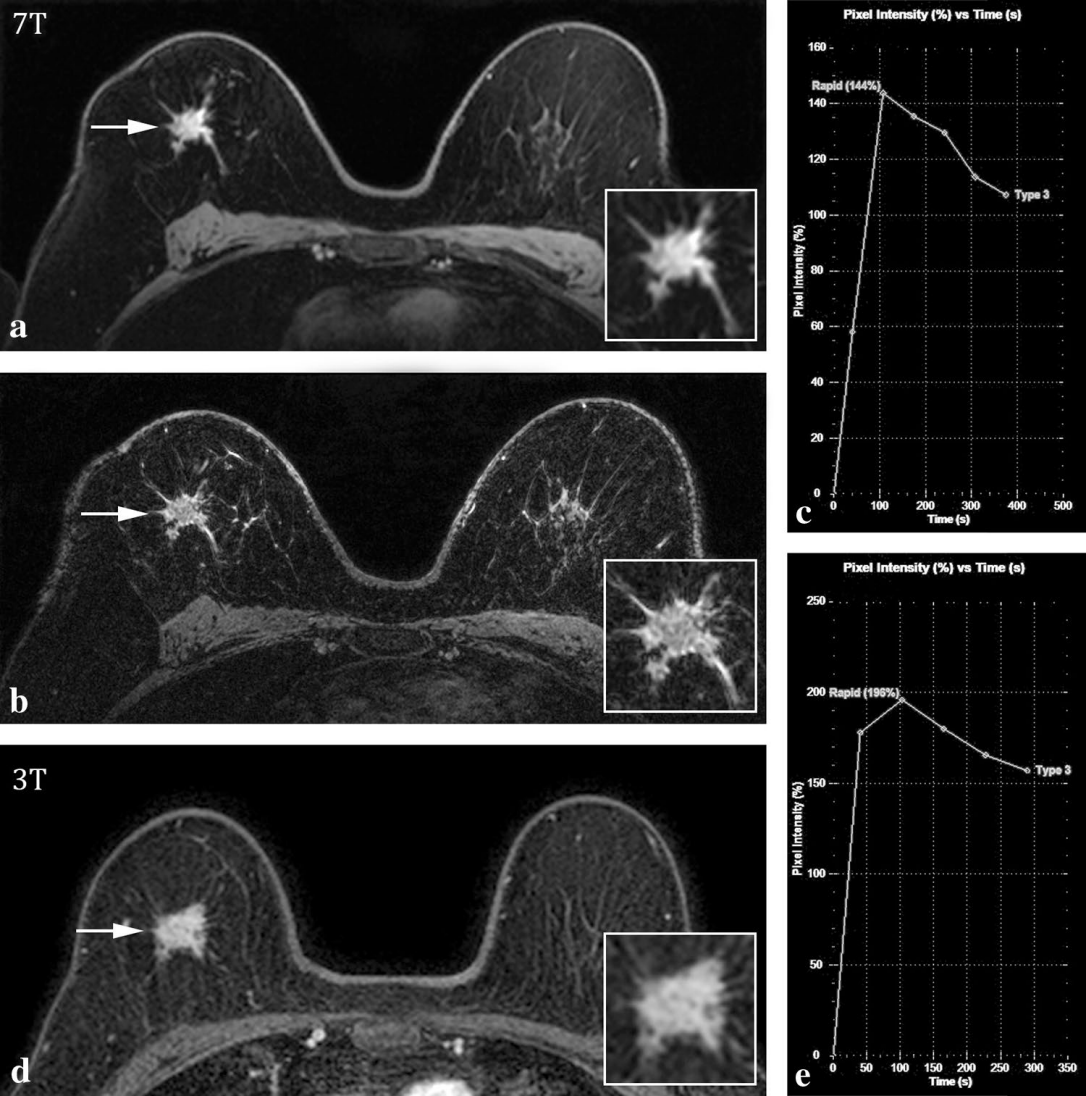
7T (a–c) and 3T (d, e) MRI images of a 67-year-old female with an invasive lobular carcinoma in her right breast. Transverse image of 2nd post contrast-injection series (a, d) shows an irregular mass lesion with spiculated margins (*arrows*) on both field strengths. *Inset* shows zoomed-in image. Ultra-high resolution 7T image of the same slice (b). The kinetic curve assessment showed an initial rapid rise and wash-out pattern in the delayed phase on both field strengths (c, e). Both observers rated the lesion as BI-RADS 5
